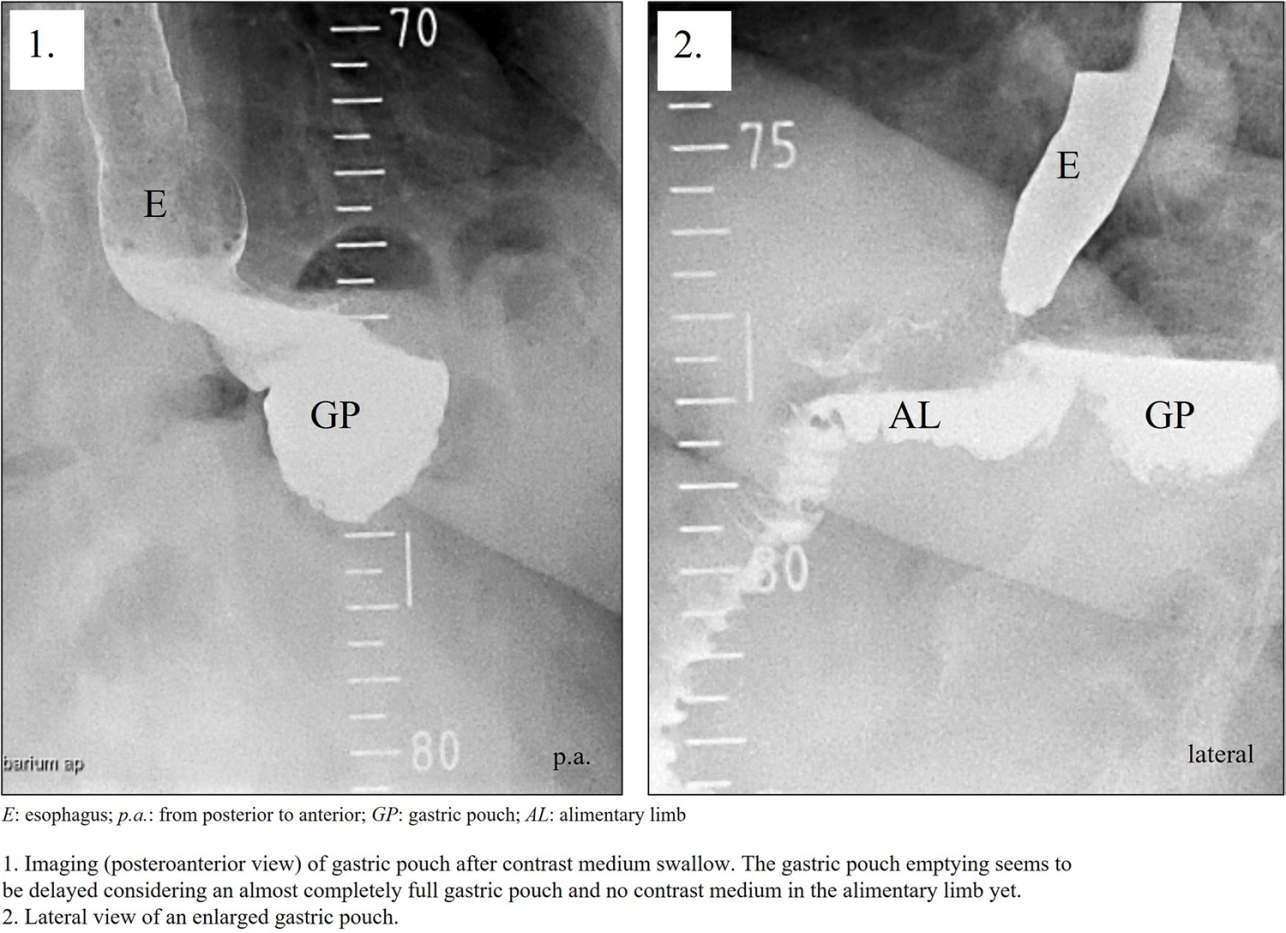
Contrast medium swallow X-ray
